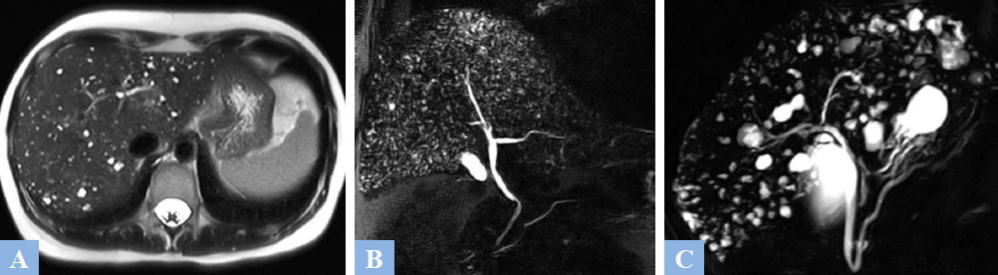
Caroli syndrome in a 47-year-old patient was discovered during an episode of cholangitis. A. T2-weighted MR image in the axial plane showing hepatic cystic lesions with T2 fluid hyperintensity, communicating with the bile ducts. B. 2D radial MR cholangiography sequences. Cystic dilations of the bile ducts (arrowhead) without stenosis at the origin of the dilations. C. T1-weighted fat-suppressed MR Image in the axial plane after gadolinium administration at the portal phase of enhancement. “Dot sign” (red circle) corresponding to the visualization of a portal vein within a hepatic cyst.
